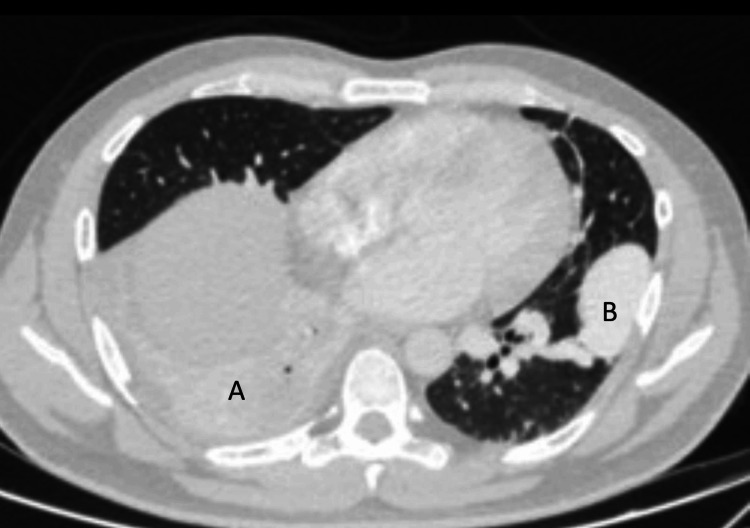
CT thorax plain (lung window) A- Collapse consolidative changes with air bronchogram and focal cavitatory lesion (3.2 x 2.4 cm) in right lower lobe, suggestive of infective etiology. B- Homogenously enhancing, well circumscribed, juxtafissural soft tissue density nodule in left lung with feeding artery as segmental branch from left lower lobar artery and draining vein as left inferior pulmonary vein. Features are suggestive of pulmonary arteriovenous malformation with nidus.
